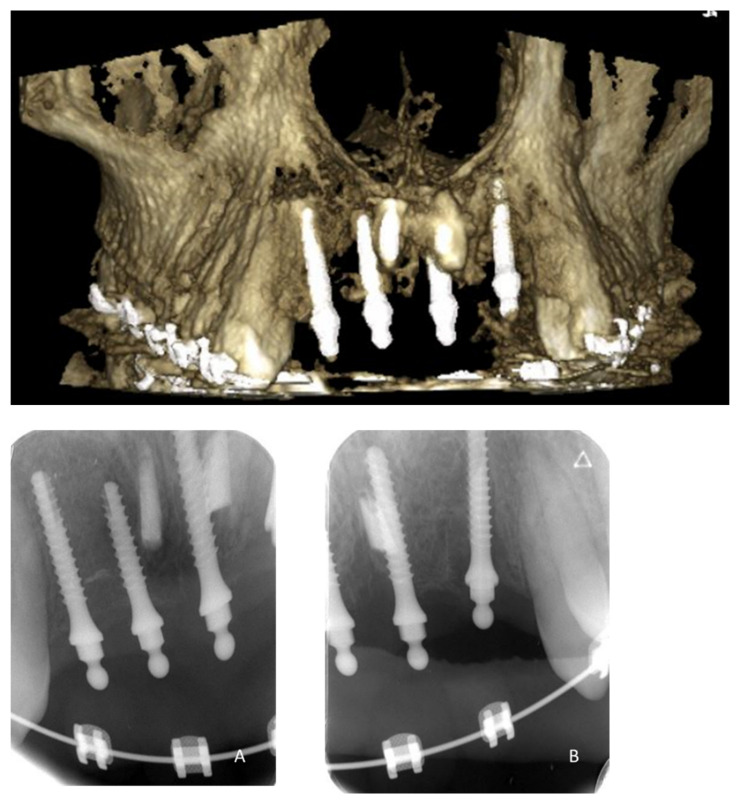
CBCT and retro-alveolar X-rays (A,B) of the 4 implants in place before the prosthetic phase.
